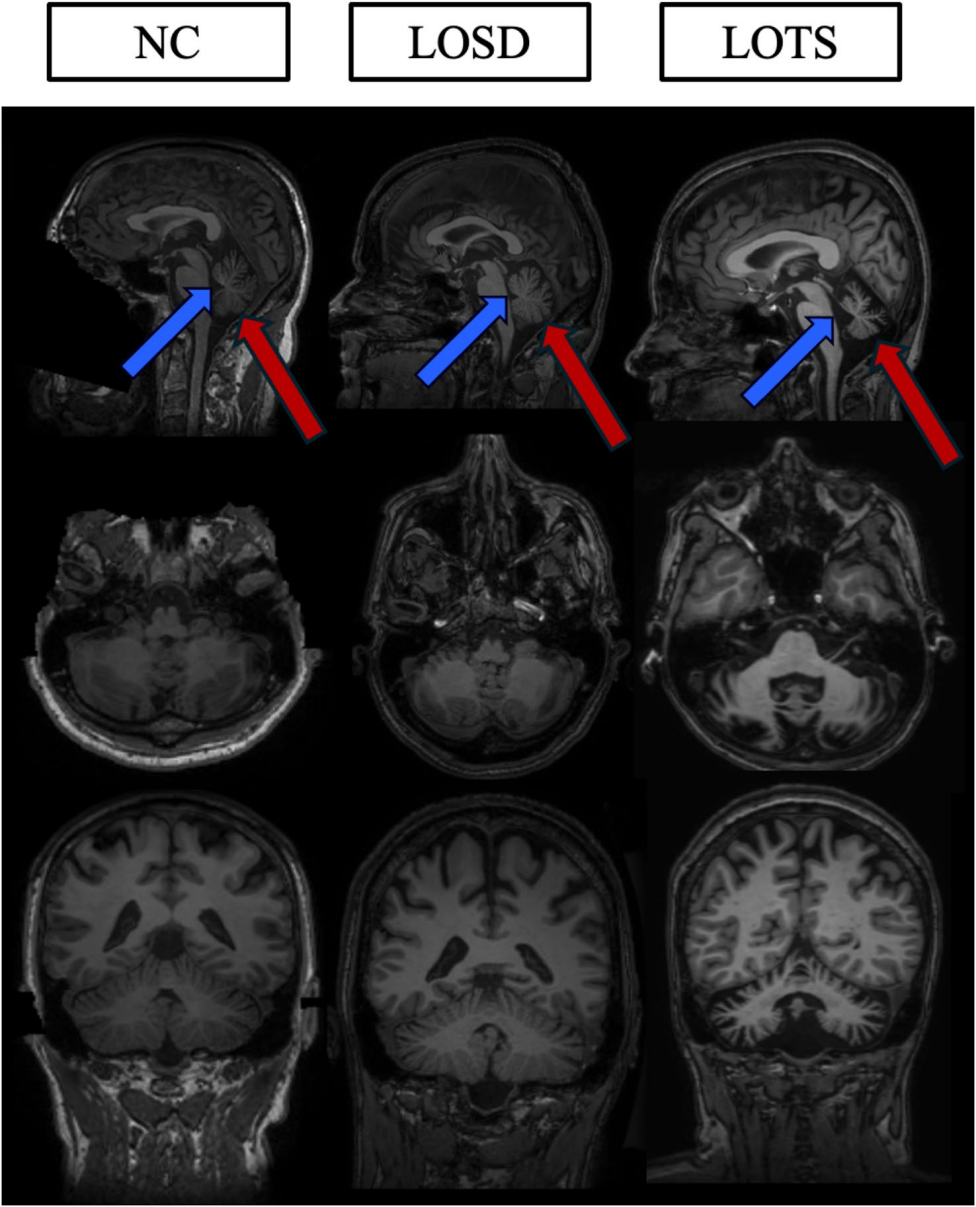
T1-weighted MRI comparisons. One age matched neurotypical control (NC, 55-year-old male), one age matched LOSD patient (56-year-old male), and one age matched LOTS patient (56-year-old female) shown in the Sagittal, Axial, and Coronal planes. The blue arrows designate the 4 th ventricle for each participant and the red arrows designate the cerebellum for each participant. The LOTS patient shows significant cerebellar atrophy in all three views. The neurotypical control was from the NIMH dataset
